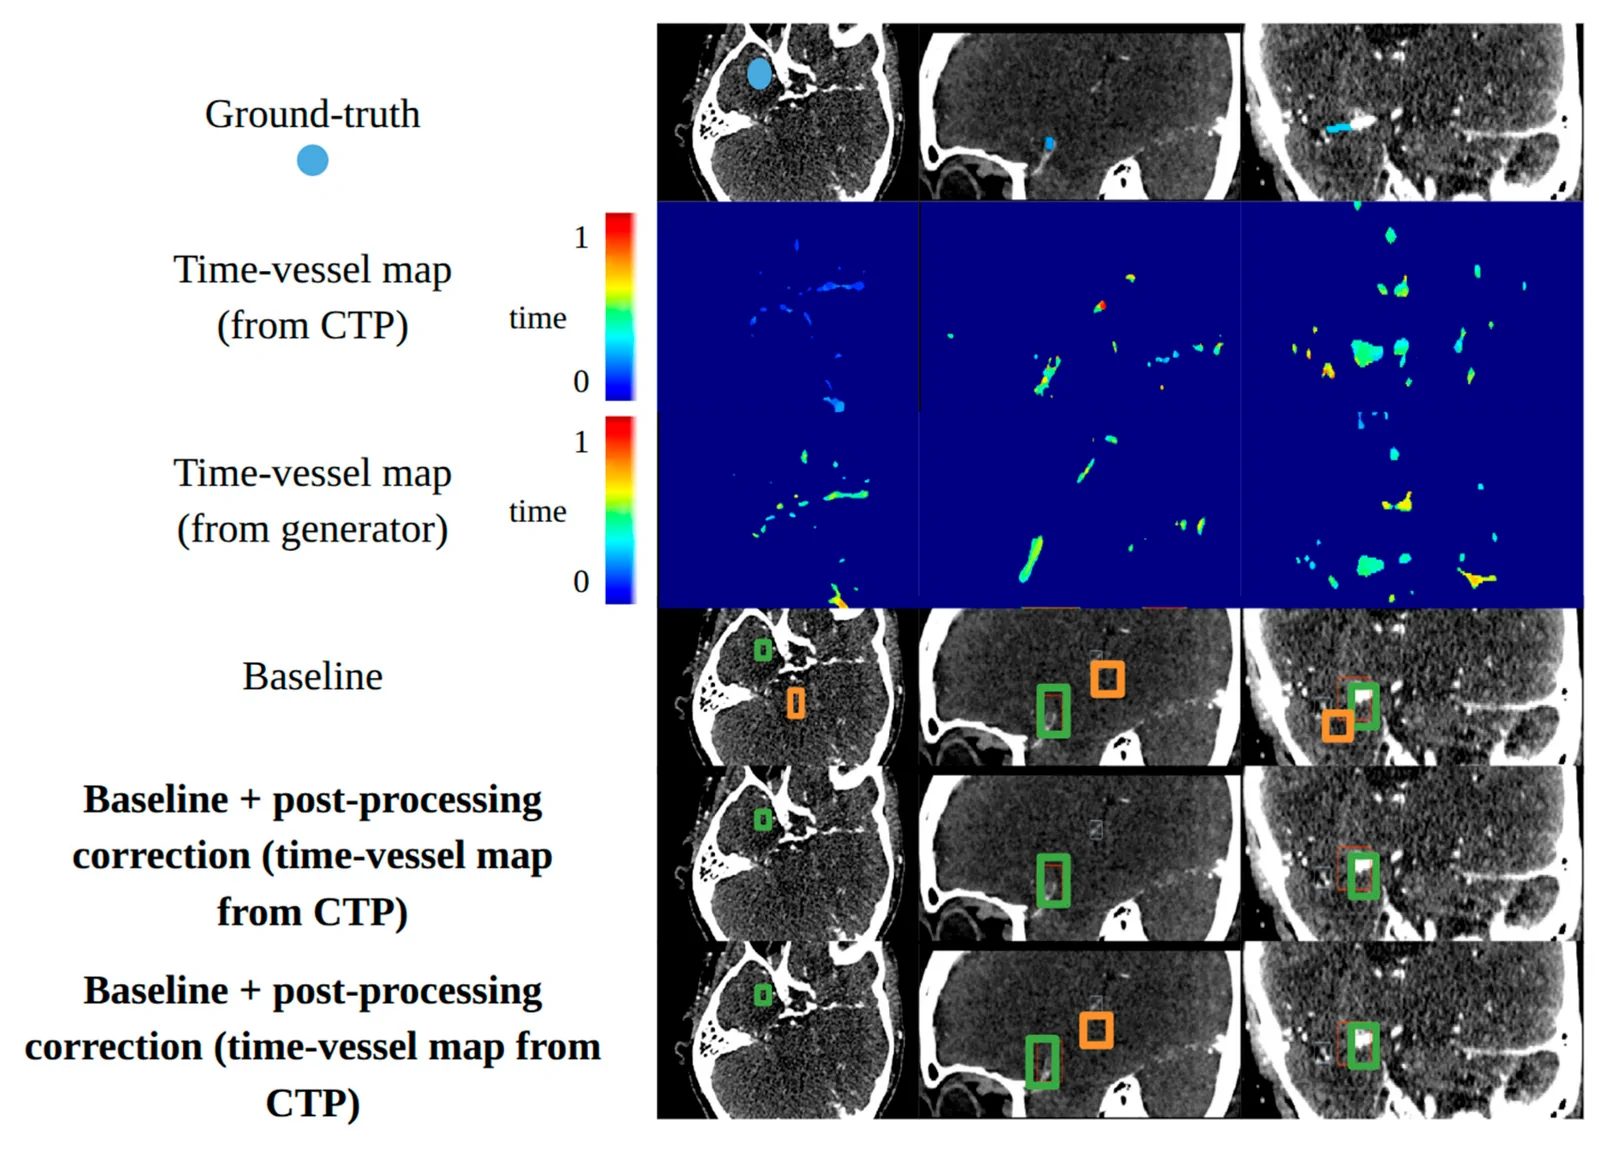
Influence of the CTA-to-time-vessel map generator in the threshold-development subset, across three cases, including CTP-derived vessel maps, generator-derived time-vessel maps, and performance for the baseline detector, with and without post-processing correction using both time-vessel map types. Blue blobs denote ground-truth occlusions; orange boxes denote incorrect occlusion detections; green boxes denote true occlusion detections. Methods in bold use the post-processing correction framework.
